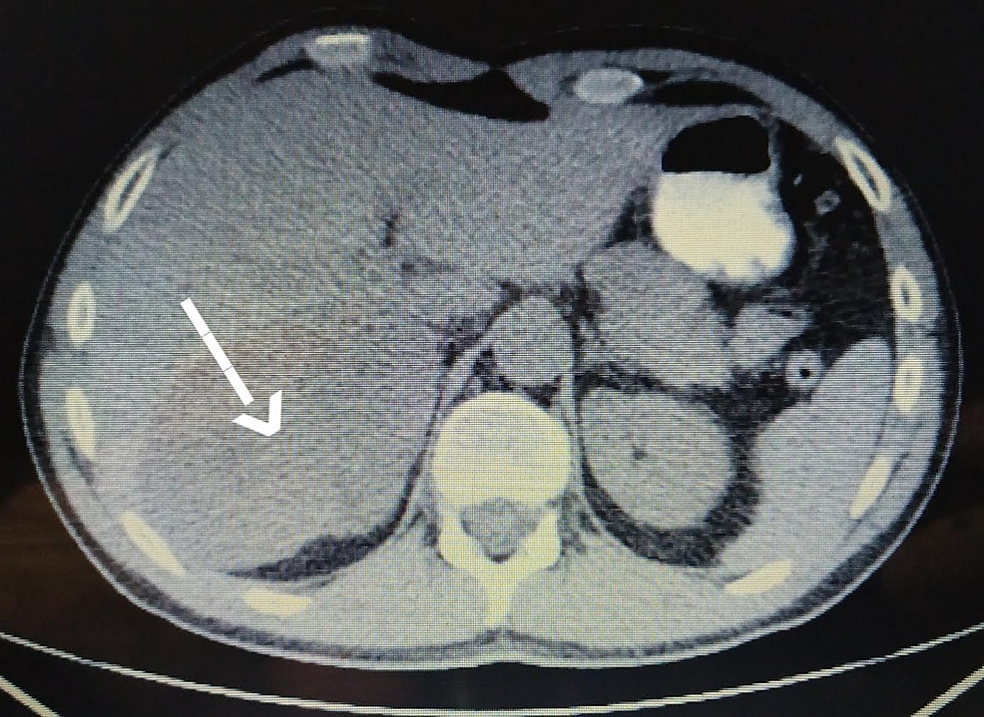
CT scan indicating an adrenal mass
